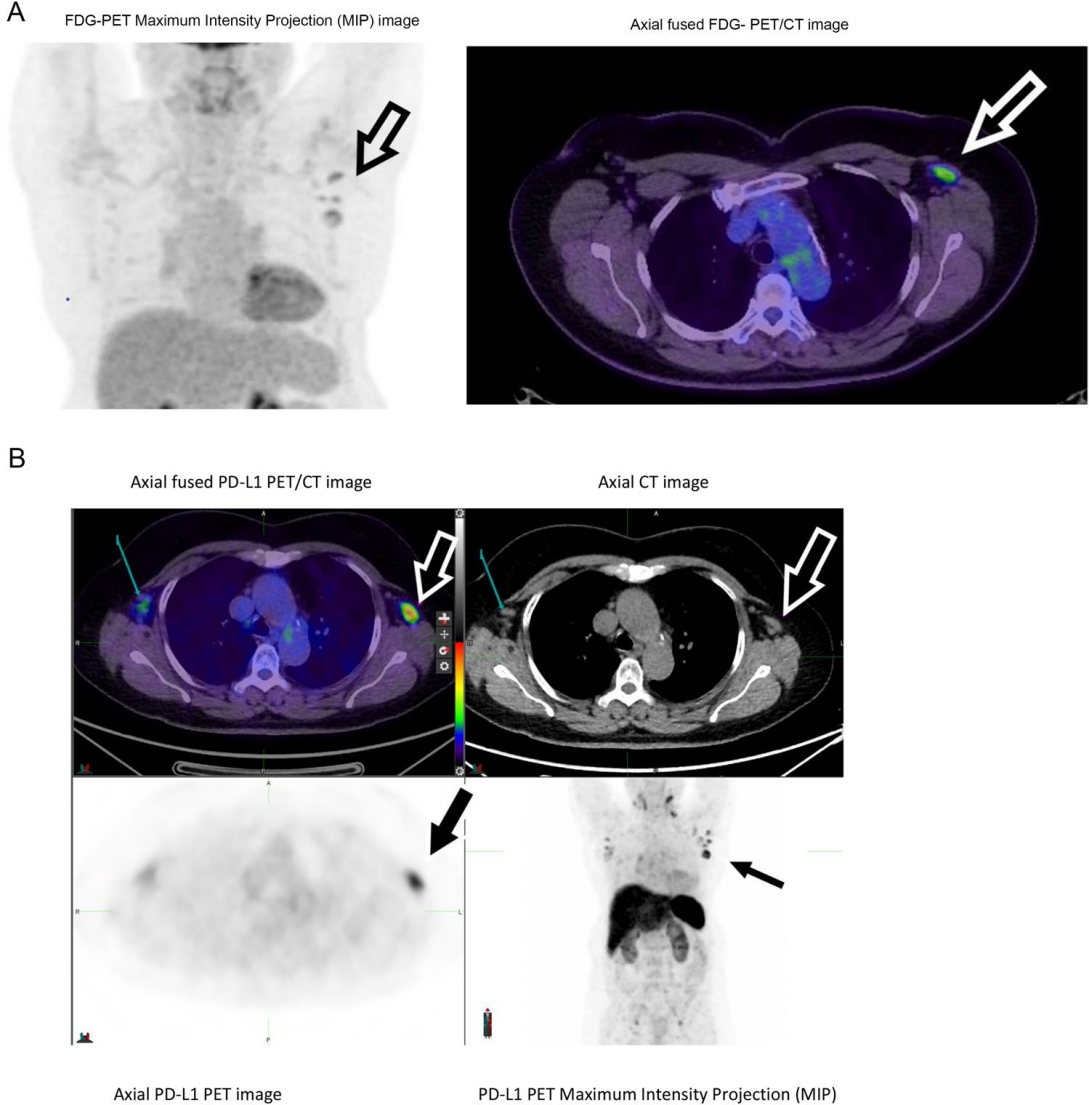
Patient 3 after COVID-19 vaccination, left deltoid muscle. (A) FDG-PET scan. Left axillary lymph nodes are indicated by arrows. (B) PD-L1 PET scan day 5. Left axillary lymph nodes are indicated by large arrows
